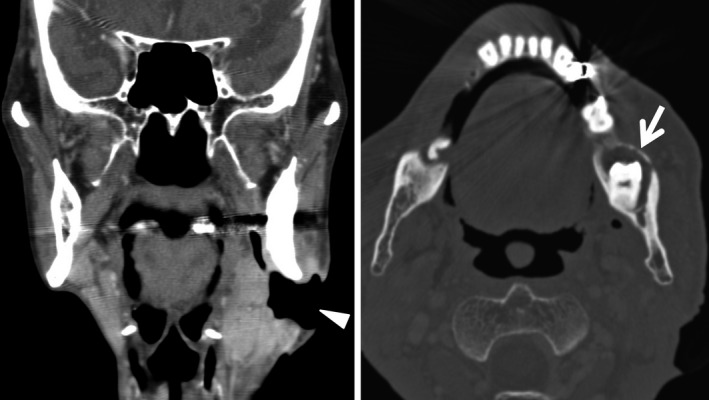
Contrast‐enhanced and bone window computed tomography images revealed cutaneous ulcer (arrow head) at left submandibular area creating a fistula continuous with left third molar pericoronitis with osteosclerosis (arrow).
